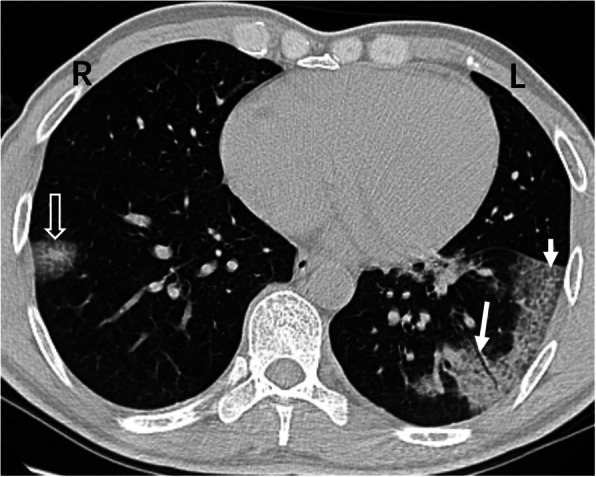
CT image of a 46-year-old male patient showing a combination of patchy GGO with consolidation (long black arrow) in the anterior basal segment of the right lower lobe, and a localized lesion in the peripheral lung field adjacent to the subpleural region. A combination of GGO with consolidation in multiple shapes is seen in the lateral and posterior basal segments of the left lower lobe, where most areas show a crazy-paving appearance (short white arrows), with visible air-filled bronchi (long white arrow) in the lesion. R = Right, L = Left
